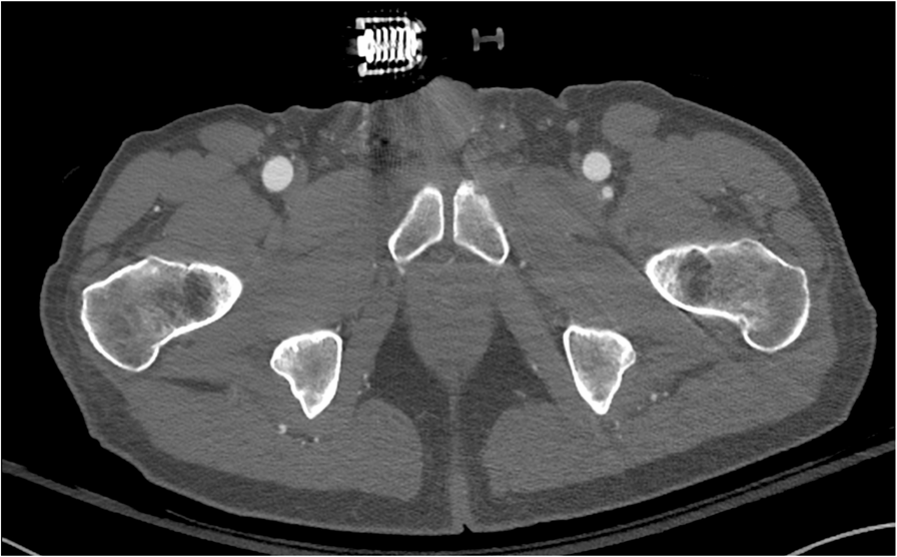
Computed tomography with binder *in situ* showing normal pubic diastasis
